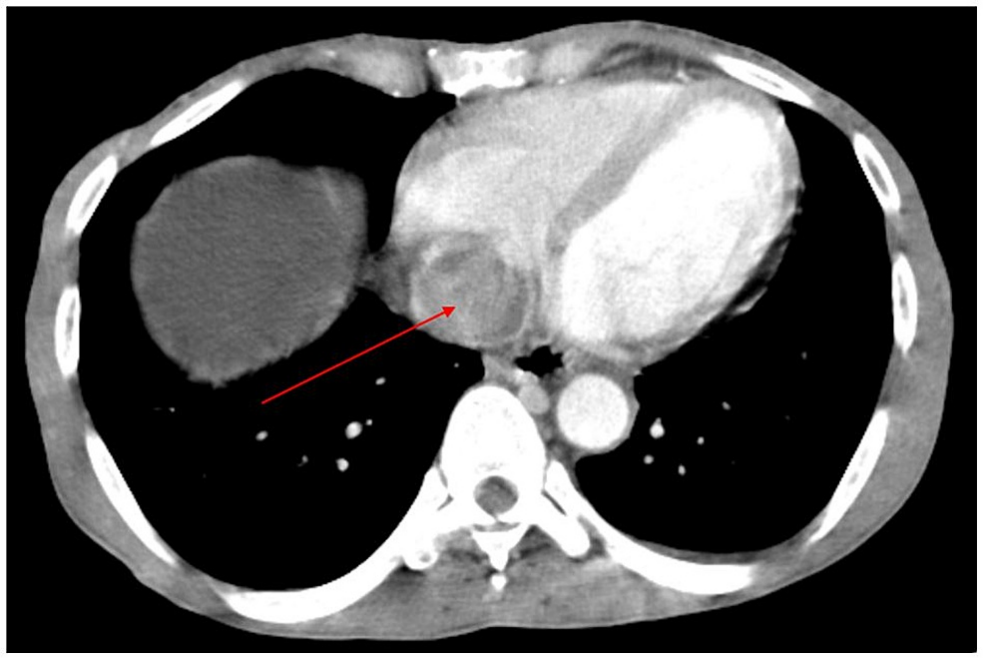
Axial post-contrast CT image through the inferior chest demonstrates tumor in the right atrium (straight arrow).
